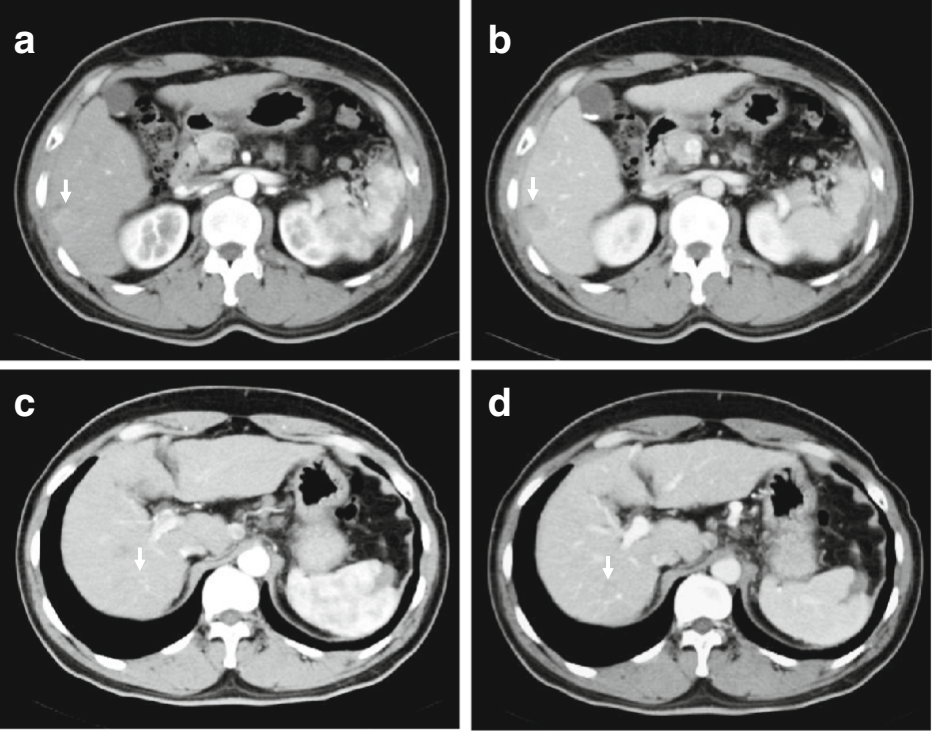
Abdominal computed tomography (CT) of the two tumors. a A 23-mm tumor had early-phase enhancement in the 6^th^ segment of the liver (*arrow*). b The tumor showed late-phase washout in the 6^th^ segment of the liver (*arrow*). c A 10-mm tumor showed slight early-phase enhancement in the 7^th^ segment of the liver (*arrow*). d The tumor showed late-phase washout in the 7^th^ segment of the liver (*arrow*)
